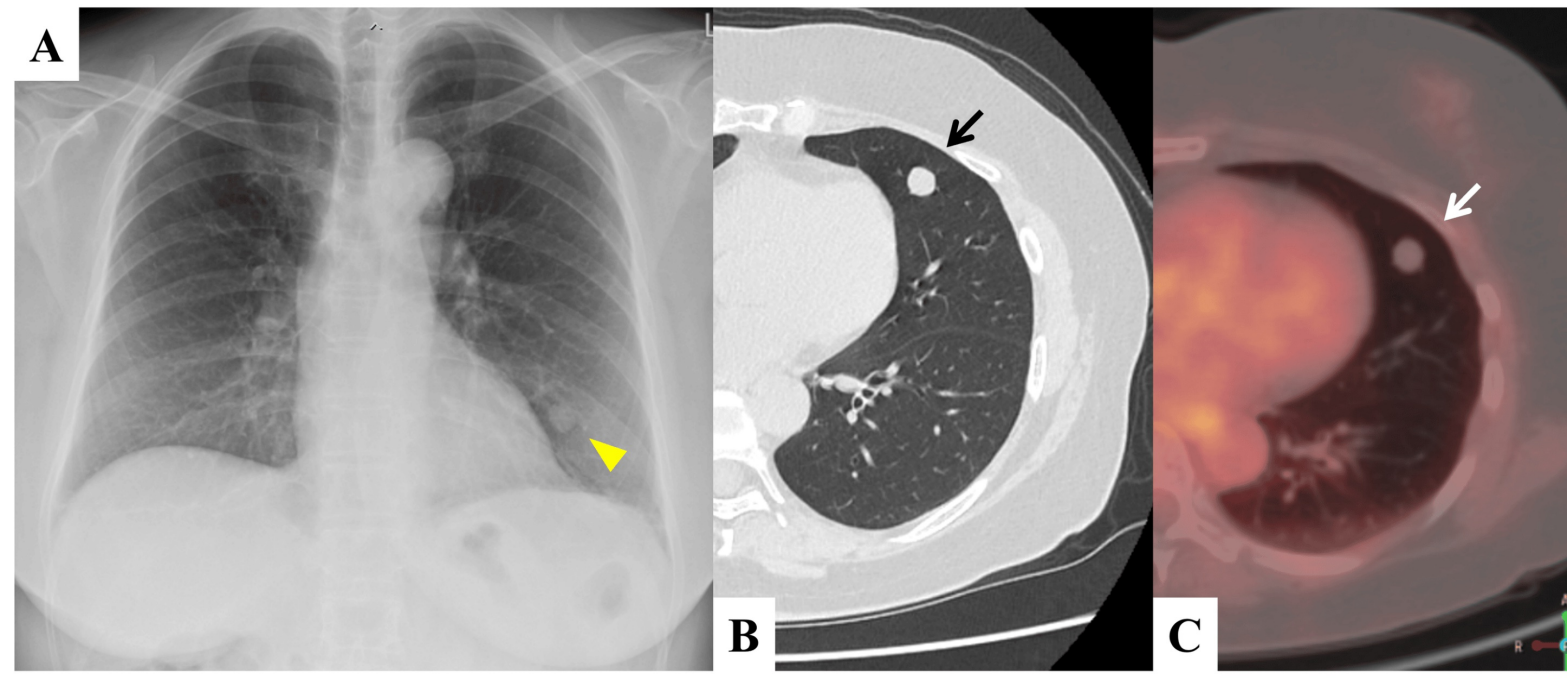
Clinical images of Case 2 (A) Chest radiograph showing a well-defined mass in the left lower pulmonary field (yellow arrowhead). (B) Chest CT showing a mass measuring 11 mm in size in the left middle lobe S5 (black arrow). (C) An 18F-FDG-PET/CT scan showing no accumulation (SUVmax=0.90) (white arrow). SUVmax: maximum standardized uptake value
